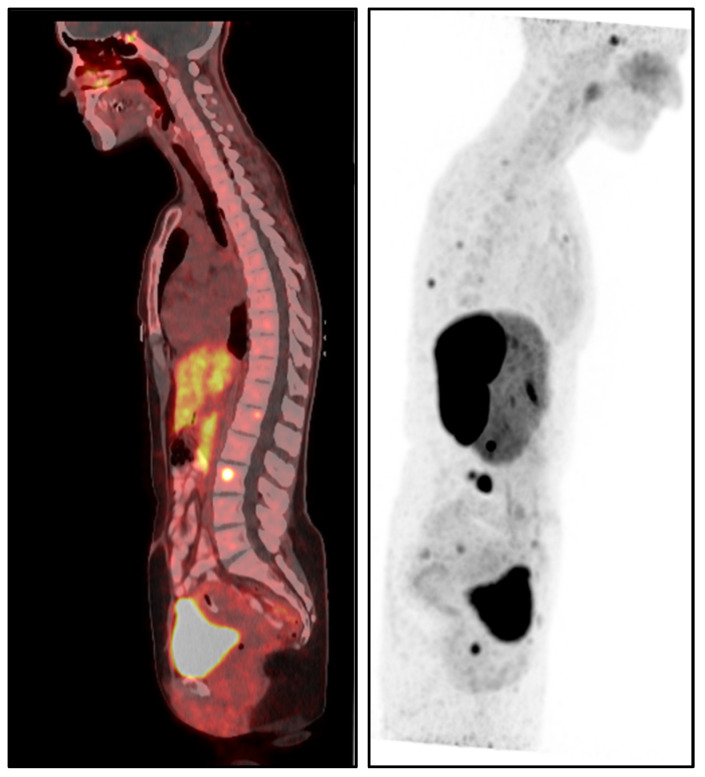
DOPA PET/CT sagittal images showing increased radiotracer uptake in the skeletal system, consistent with skeletal metastases from metastatic pheochromocytoma. The images demonstrate significant involvement in the spine and pelvis.
